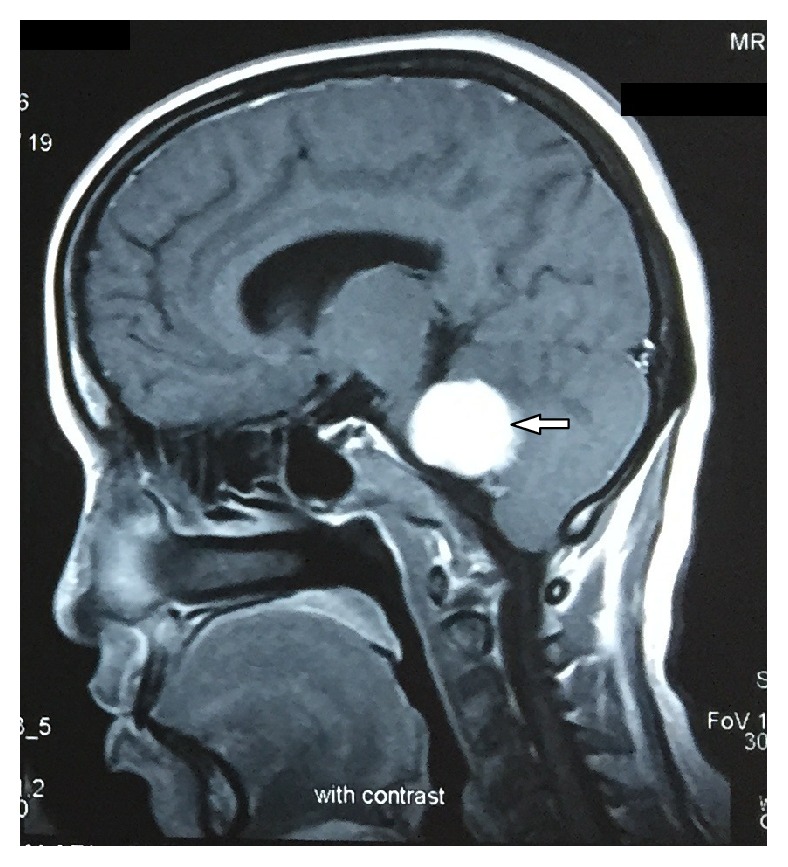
Contrast sagittal MRI shows well-defined heterogeneously enhancing T1 hypo and T2 hyperintense mass in the right cerebellopontine angle causing mild mass effect on the brain stem (white arrow).
